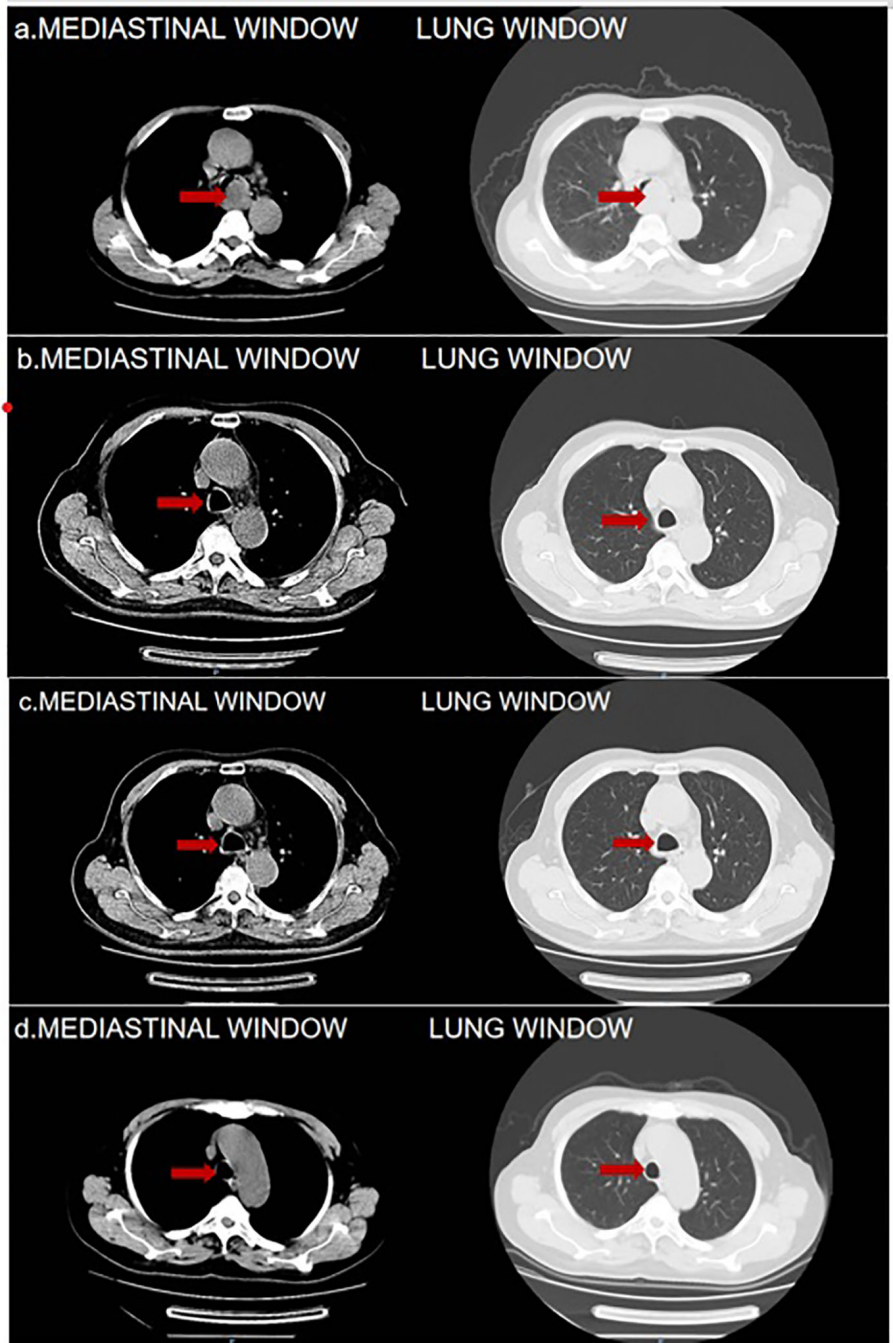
(A) 2023-5-2 Pulmonary CT Findings: Tracheal Prominence Occupancy. (B) 2023-6-28Pulmonary CT Findings: Significant Reduction in the Mass Below the Tracheal Prominence. (C, D) 2023-8-22 and 2023-11-1Pulmonary CT Findings: Absence of Mass Below the Tracheal Prominence in Both. The red arrows are used to indicate the location of tumor.
